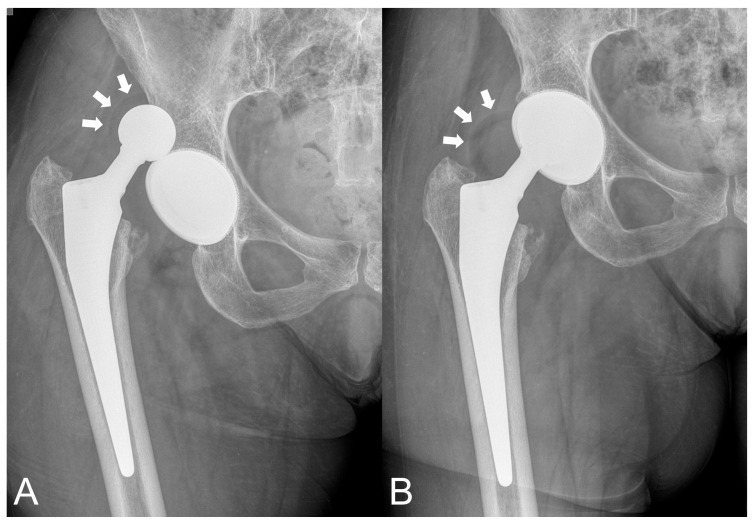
Early IPD case occurred 2 months after modular DM THA during unsuccessful hip closed reduction to treat a dislocation caused by a traumatic event. (A): Plain radiograph taken after dislocation, showing outside articulation the mobile polyethylene liner correctly assembled over the 28 mm ceramic head (white arrows). (B): Plain radiograph taken immediately after the hip closed reduction attempt, showing the IPD with the mobile polyethylene liner dissociated from the small head, the so-called “bubble sign” (white arrows). Polyethylene liner dissociation is likely due to the great traction forces during the closed reduction maneuver which are higher and overwhelm the push out force of the retentive mobile polyethylene liner.
